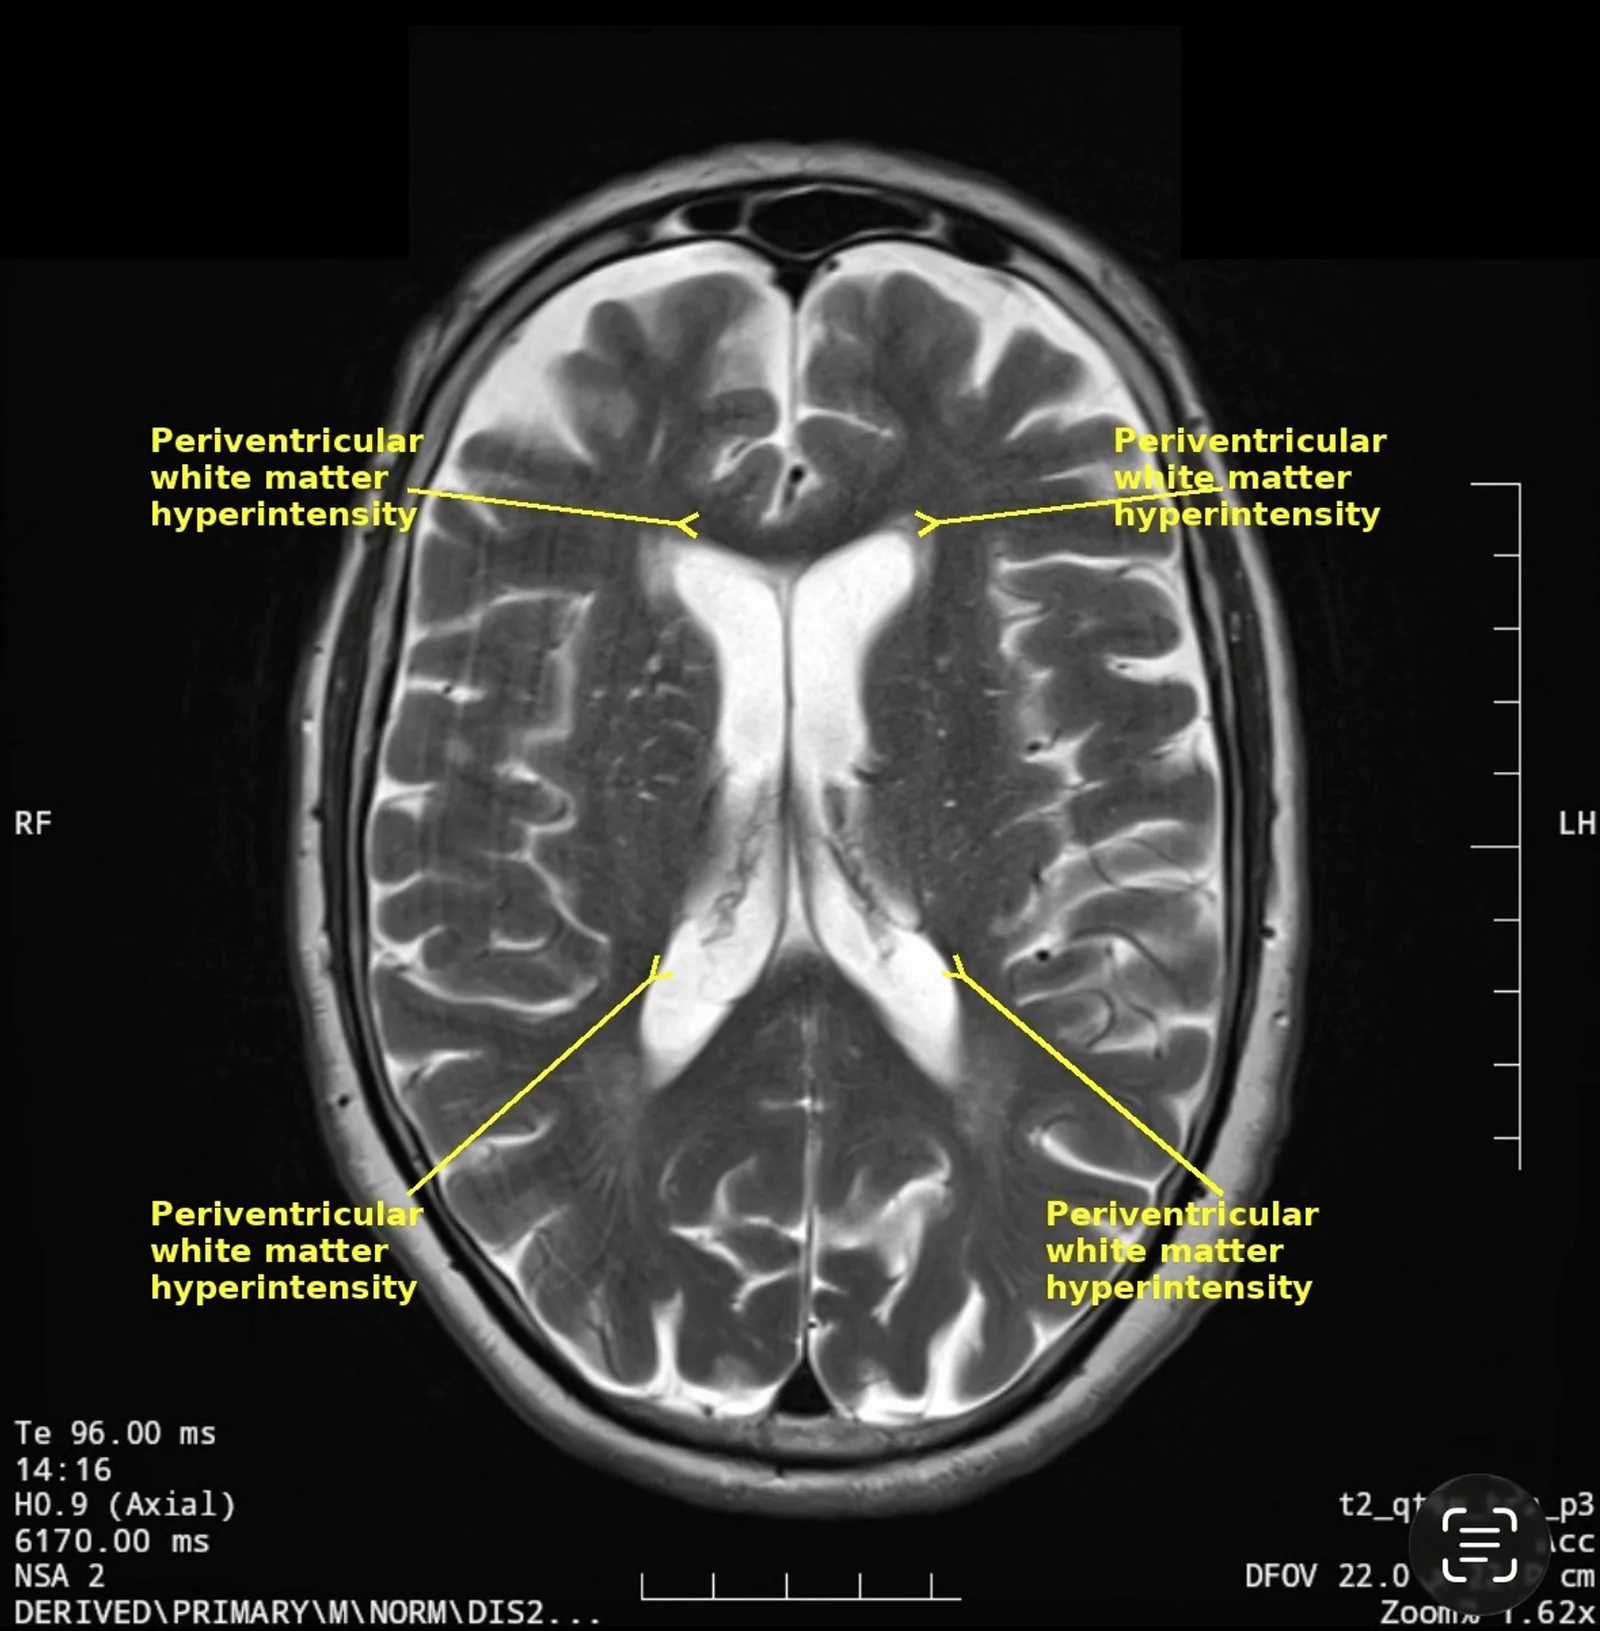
Axial T2-weighted MRI of the brain The image shows diffuse cerebral atrophy with periventricular white matter hyperintensities consistent with Fazekas grade 2 changes. No acute infarct, hemorrhage, or focal lesion is identified MRI: magnetic resonance imaging
